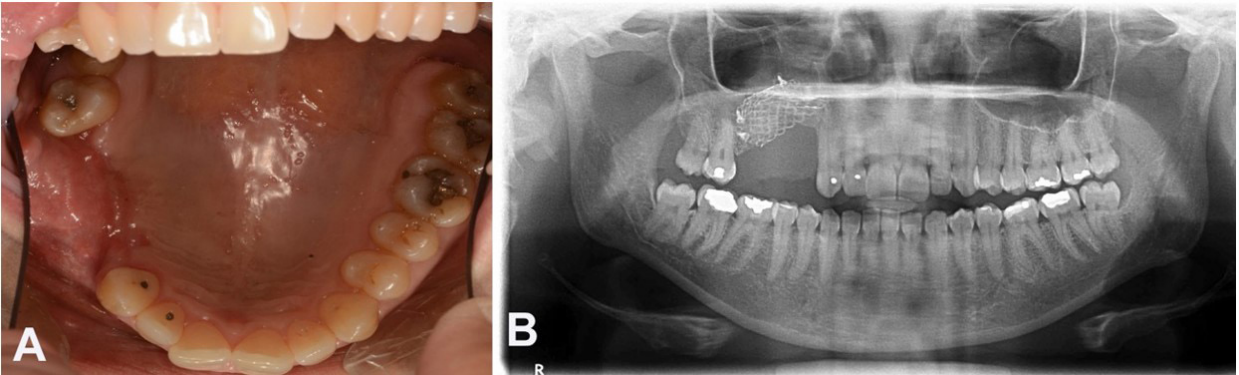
Follow-up images after thirteen months of the lesion removal. A – Intraoral view; B – Panoramic radiograph showing upper titanium mesh in position and no recurrence signs.
